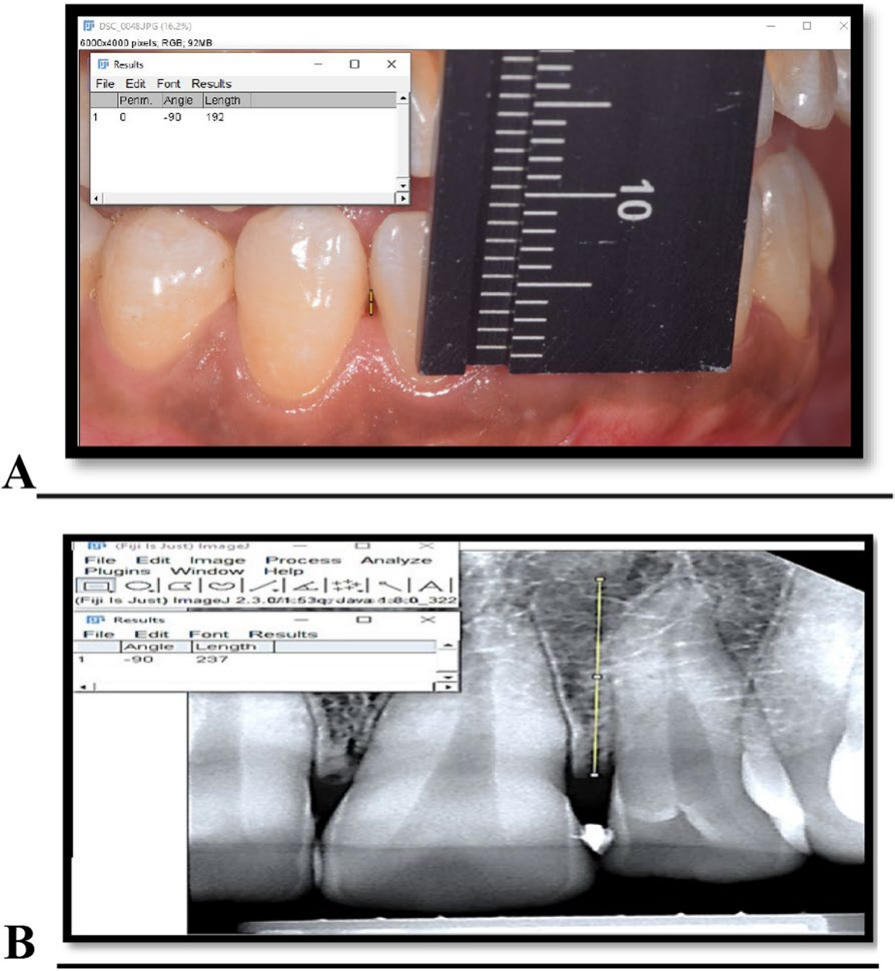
Photographic analysis (A) and Radiographic analysis (B)
